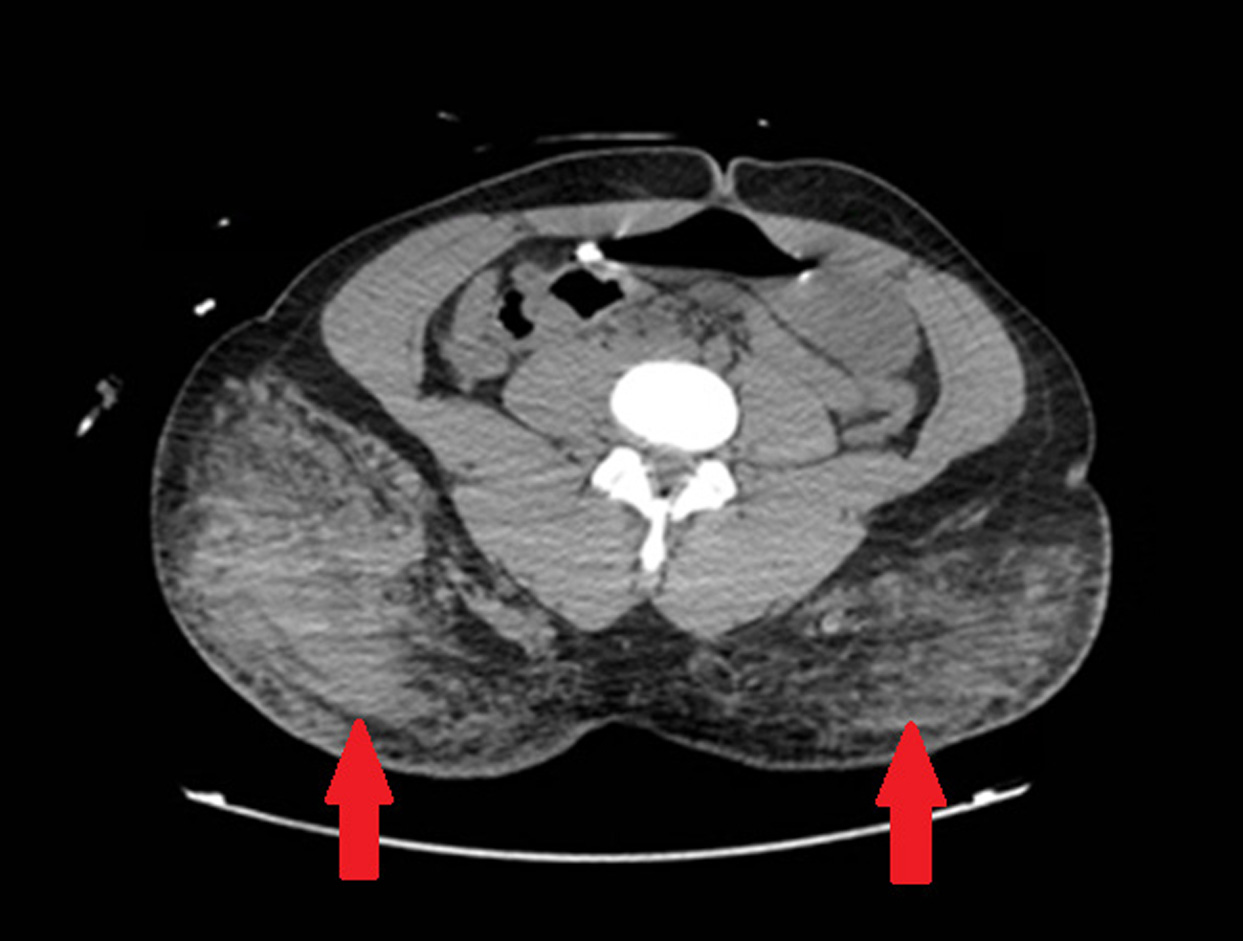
Computed tomography of the abdomen and pelvis with contrast demonstrating opacities in gluteal subcutaneous fat, suggestive of inflammation from silicone injections (arrows).
